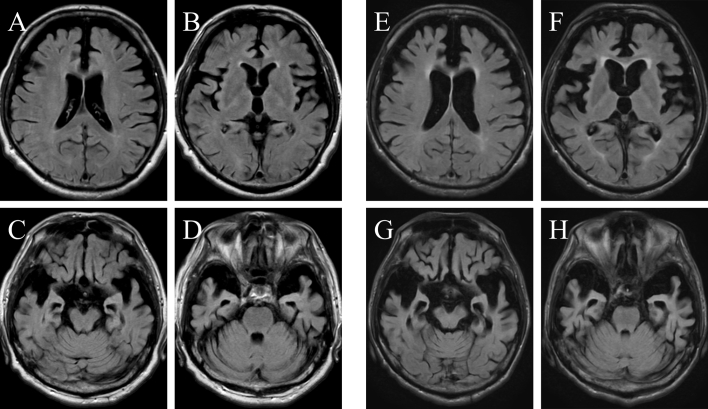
Horizontal T1-weighted brain MR images at age 53 (A-D) and age 58 (*E*-H), 6 and 11 years after onset, respectively. The images show progression of the marked atrophy of the bilateral frontal lobes (A, B, E, F), and the temporal lobes including hippocampus (C, D, G, H). The involvement of white matter is mild and brainstem regions, such as the pons and midbrain, are relatively preserved.
